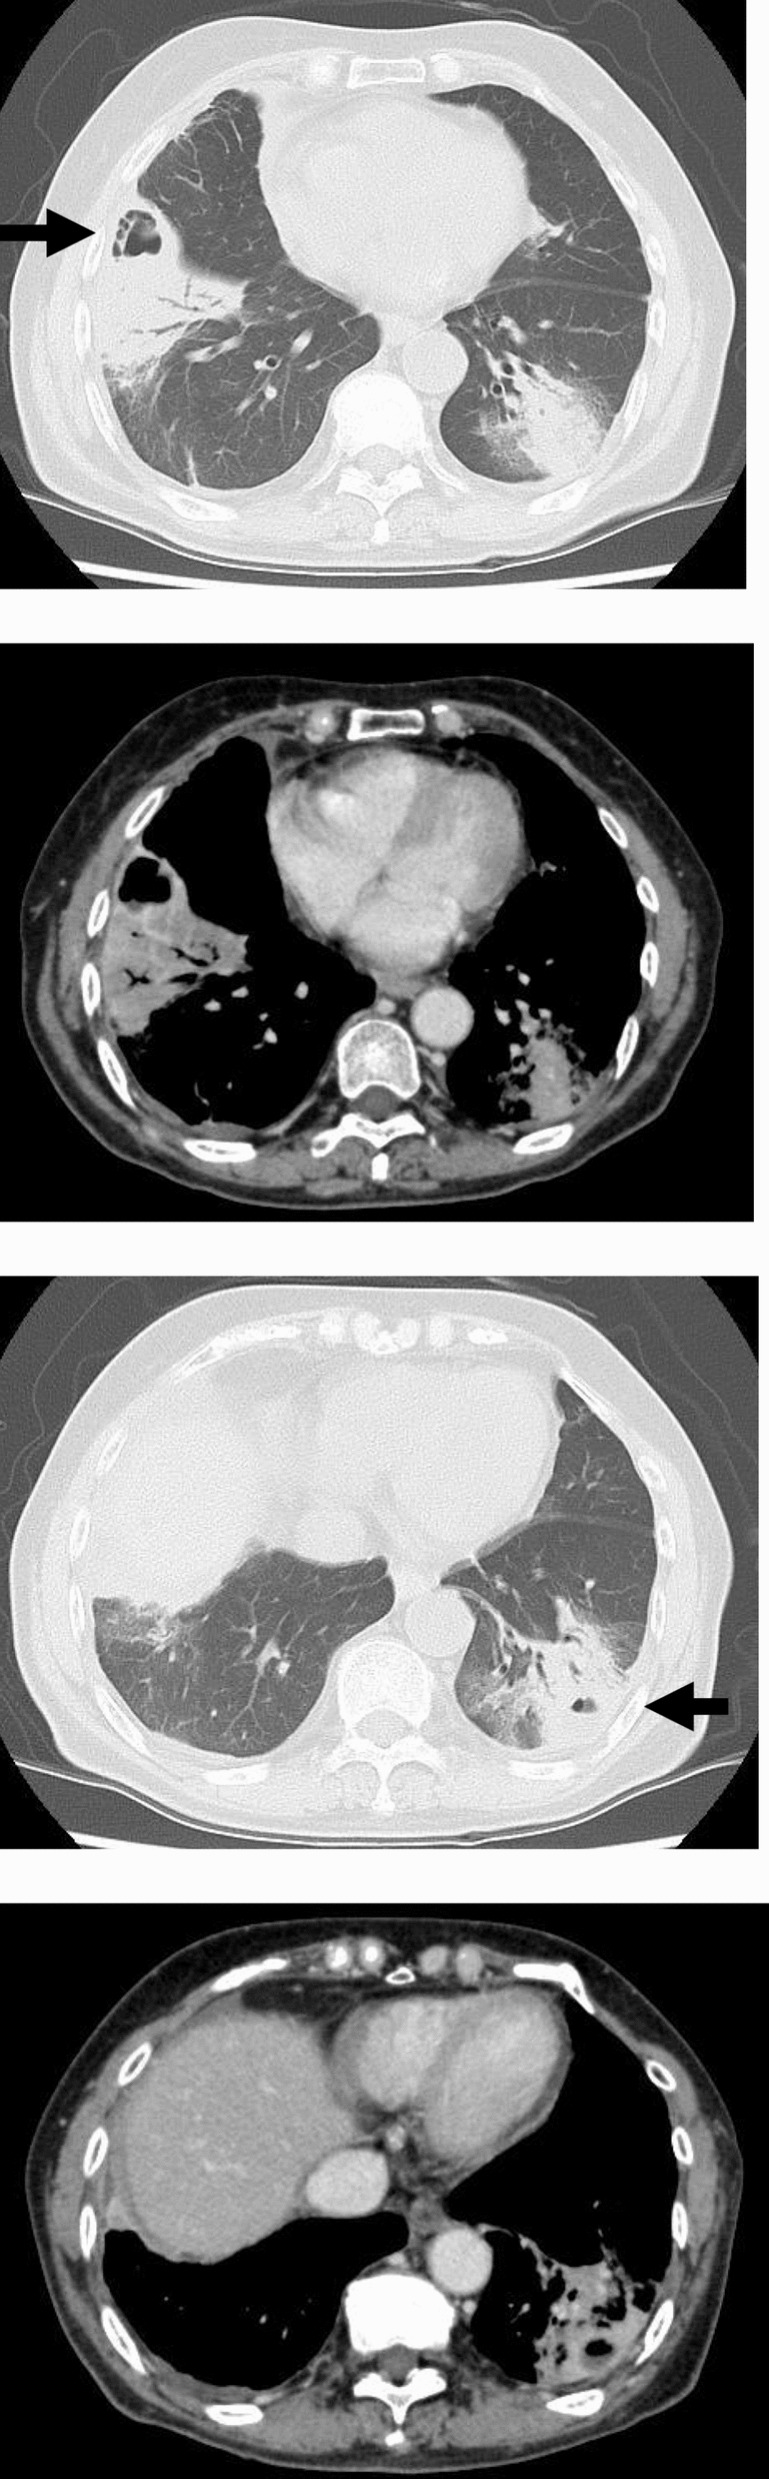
Computed tomography at presentation showing multiple lung abscesses (black arrows)
